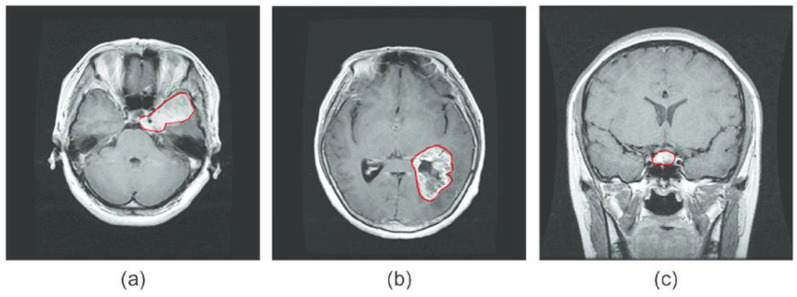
MRI images of three categories (a–c) (glioma, pituitary, and meningioma).
